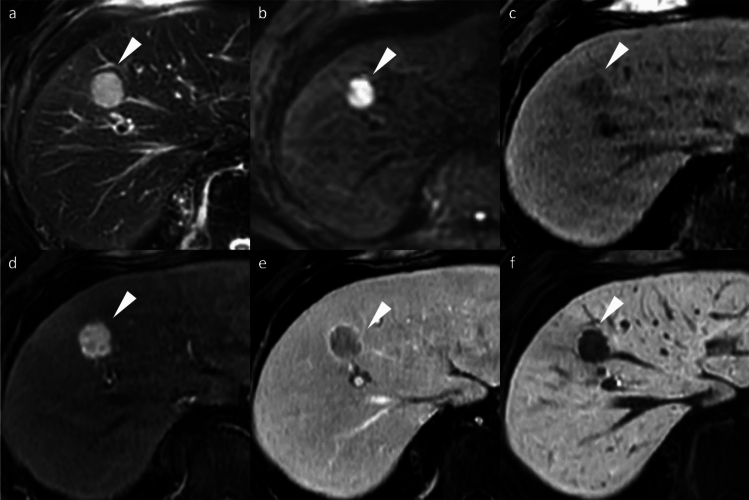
Hepatocellular carcinoma in a 79-year-old man, showing typical imaging findings. A nodule (arrowheads) located in segment 4 appears clearly hyperintense on fat-suppressed T2-weighted images (a) and demonstrates diffusion restriction on diffusion-weighted images (b). The nodule displays hypointensity on pre-contrast image (c), non-rim arterial phase hyperenhancement (d) with portal venous washout (e), and clear hypointensity on the hepatobiliary phase (f). The nodule was pathologically proven to be hepatocellular carcinoma following surgical resection
